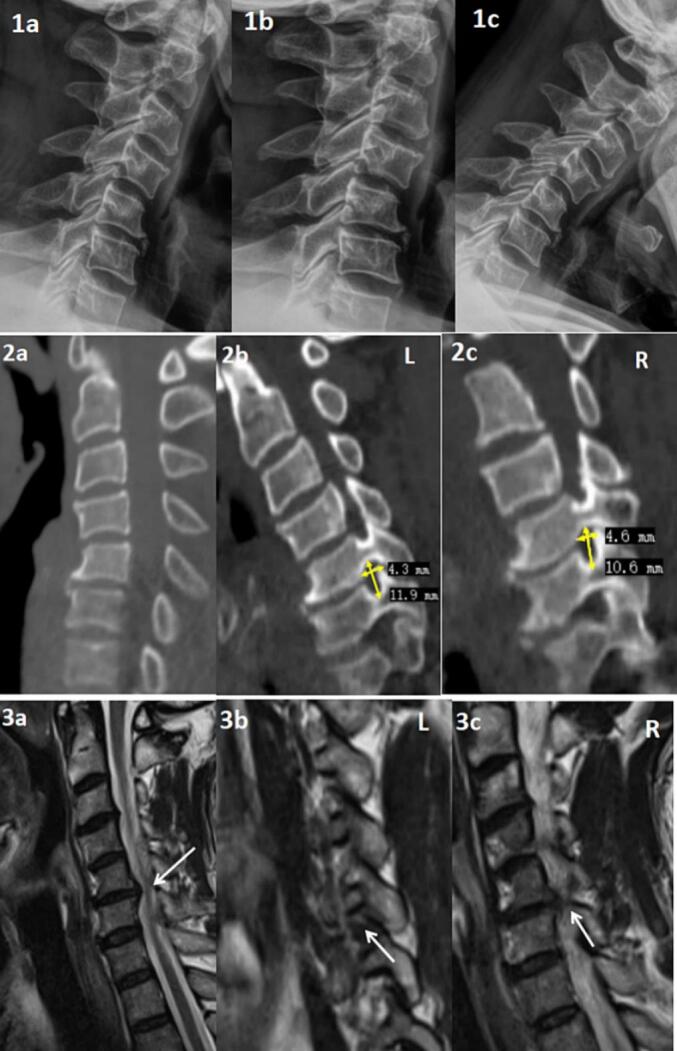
Preoperative Imaging (1a-c) Preoperative X-rays:(1a) Anteroposterior view(1*b*)Extension view(1*c*)Flexion view.(2a-c)Preoperative CT:(2a)cervical kyphosis.(2b) stenosis of the left foramen with a height of approximately 11.3 mm and a width of 4.3 mm.(2c)stenosis of the right foramen with a height of approximately 10.9 mm and a width of 4.6 mm.(3a-c)Preoperative MRI:(3a)White arrows indicate mild spinal cord compression at the C5/6 level.(3b) White arrows indicate stenosis of the left foramen at the C5/6 level.(3c) White arrows indicate stenosis of the right foramen at the C5/6 level.
